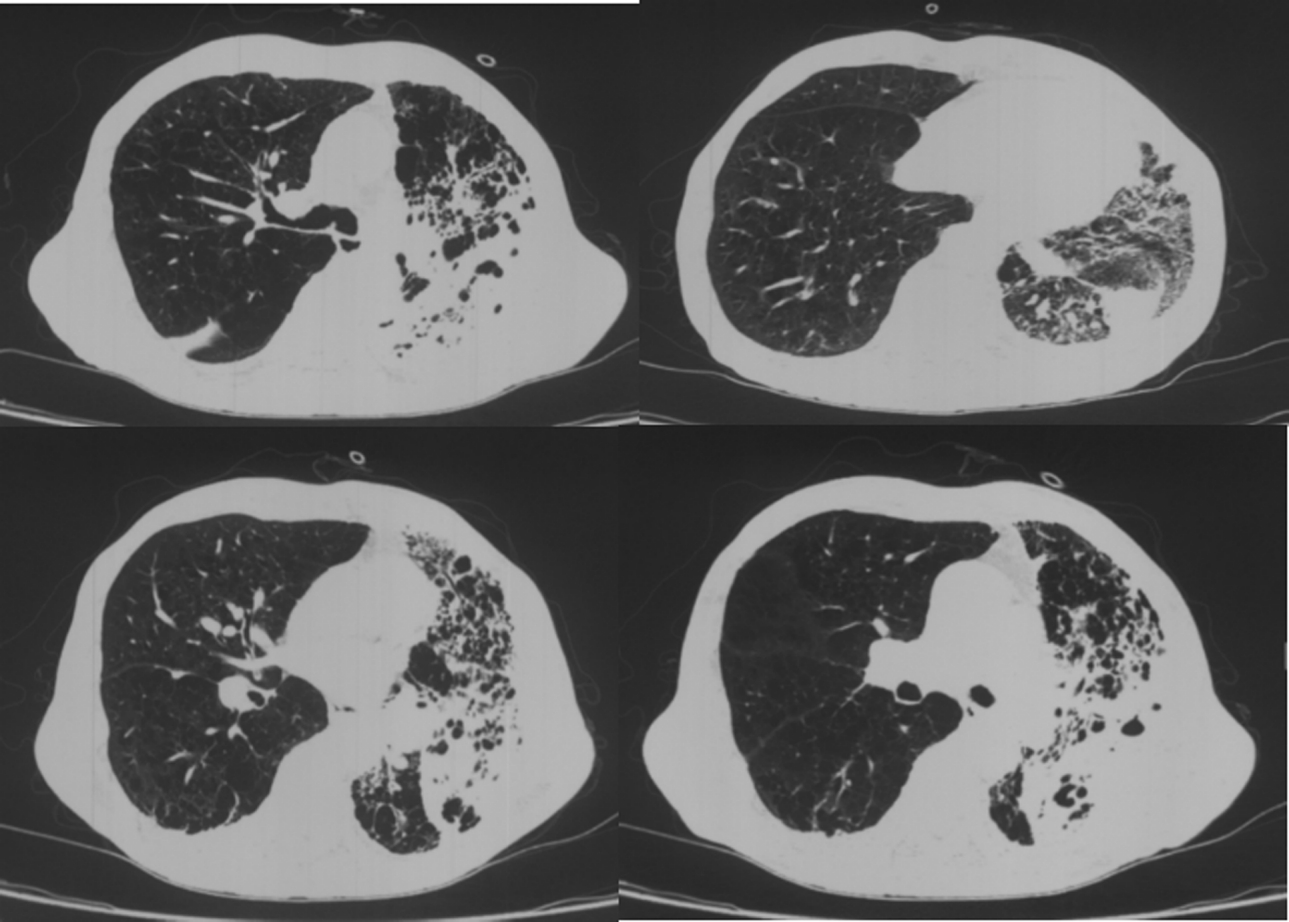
Patchy high‐density shadow in the left lung on chest CT, with an increased extent compared to the previous examination. Bilateral pleural effusion, newly appeared compared to the previous examination. Local narrowing of the left lower lobe bronchus and a suspicious soft tissue mass‐like shadow.
